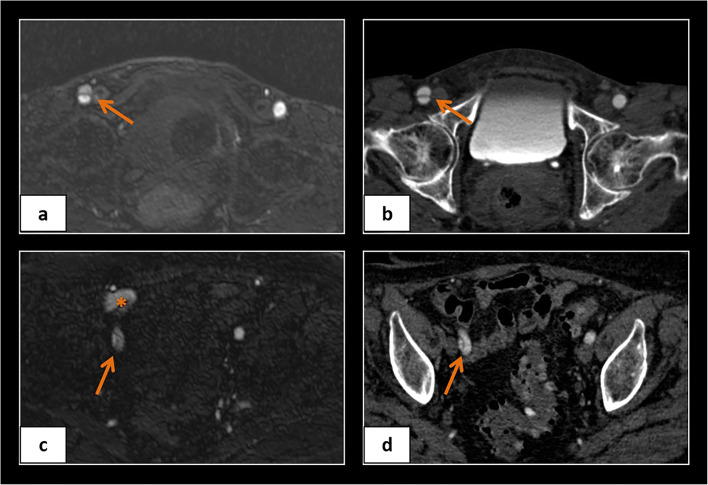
Examples showing dissection of the right common femoral artery in QISS-MRA (a) and CTA (b) and of the right external iliac artery in QISS-MRA (c) and CTA (d). The asterisk in (c) indicates artifacts due to intestinal gas
